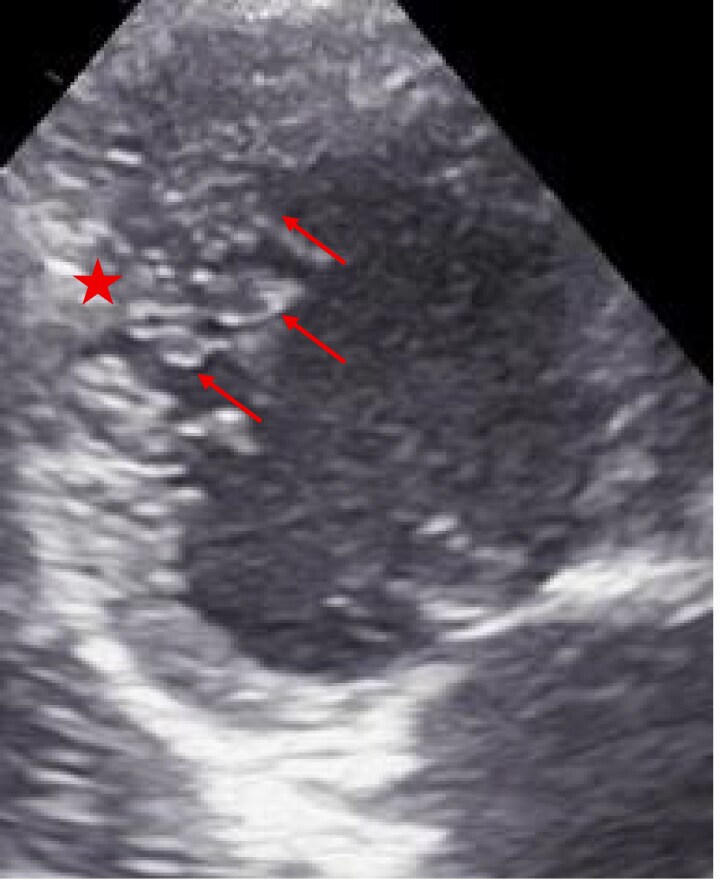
Apical long-axis view from transthoracic echocardiography demonstrating prominent trabeculations and deep intertrabecular recesses within the left ventricular apex, consistent with morphological features of left ventricular non-compaction. Red arrows highlight the regions of prominent trabeculation. The red asterisk indicates the compact myocardial layer.
